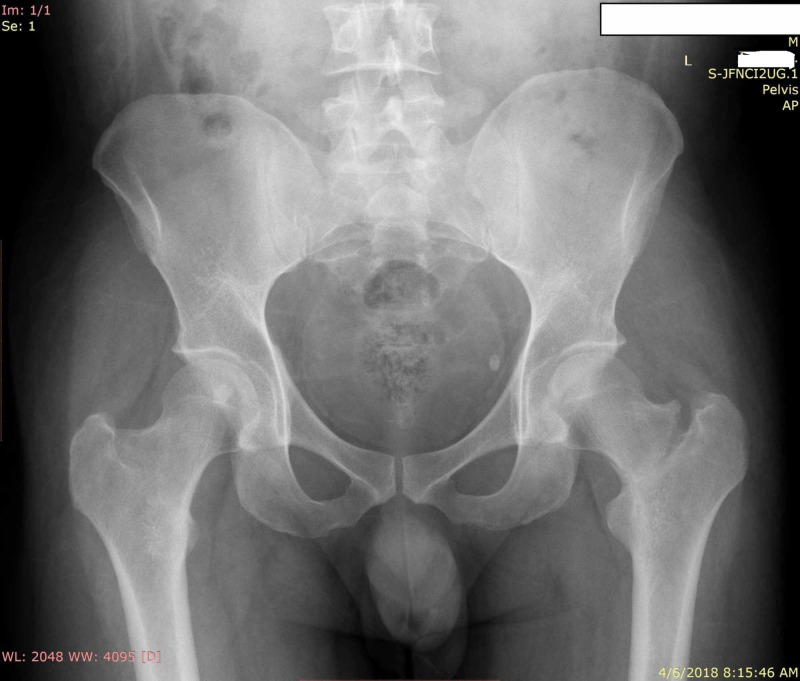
X-ray of the pelvis with both hips showing displaced basicervical fracture of left neck of femur.
